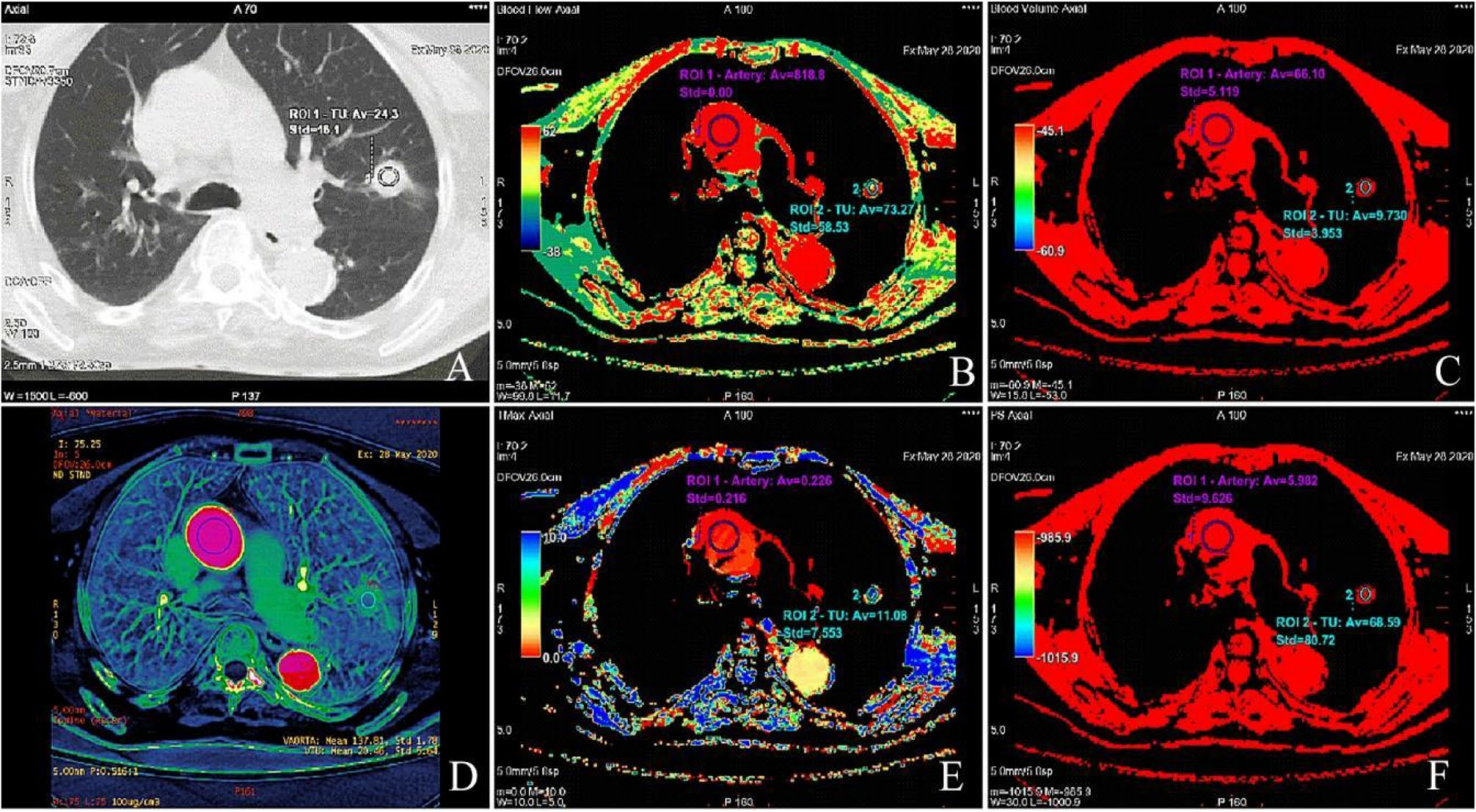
(A) CT scan displaying a circular ROI manually placed on the TU with average value of 24.3 HU. (B) BF 73.27 ml/100 g/min presents the mean flow rate through vasculature in the ROI tissue region of the TU. ROI 1 (purple color) represents the aorta, ROI 2 (green) denotes the tumor cross-section. (C) BV 9.73 ml/100 g presents the mean volume of flowing blood within a vasculature of the tissue region. (D) Contrast enhancement DECT reconstruction performed with Color maps French software on GE healthcare Workstation 4.7. (E) Tmax 11.08 s represents the delayed arrival of contrast bolus. (F) PS 68.59 ml/100 g/min denotes the total flux from the plasma to the interstitial space. *CTP* perfusion computed tomography, *ROI* region of interest, *TU* tumor, *BV* blood volume, *BF* blood flow, *Tmax* the time to the maximum of the residue function.
